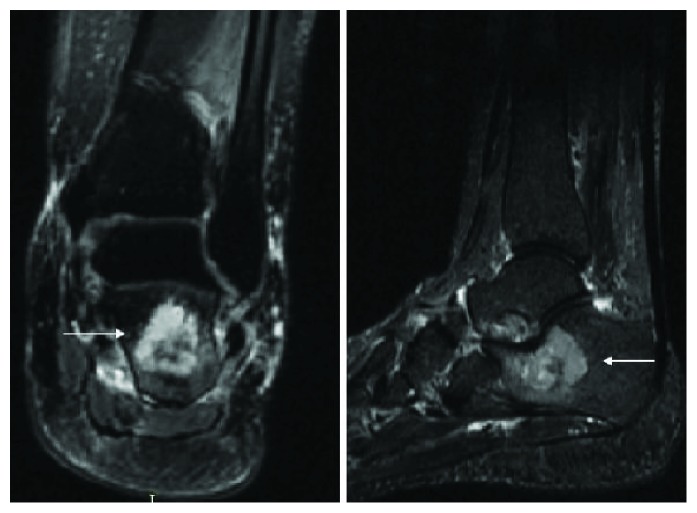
MRI of the left ankle: T2-WI with fat saturation sequences obtained in the coronal and sagittal planes confirm the presence of anterior calcaneal bone tumor (white arrows).
